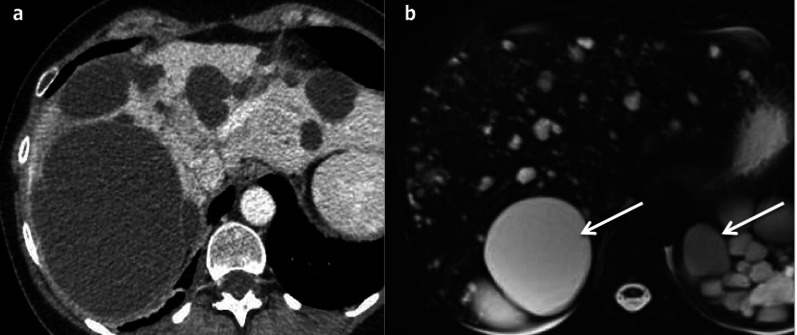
Autosomal dominant polycystic kidney disease in a 38-year-old male patient. a Axial computed tomography on portal venous phase and b axial T2-weighted magnetic resonance imaging show several simple hepatic cysts and also numerous kidney cysts (arrows), with fluid signal (hypodense/hyperintense on T2)
